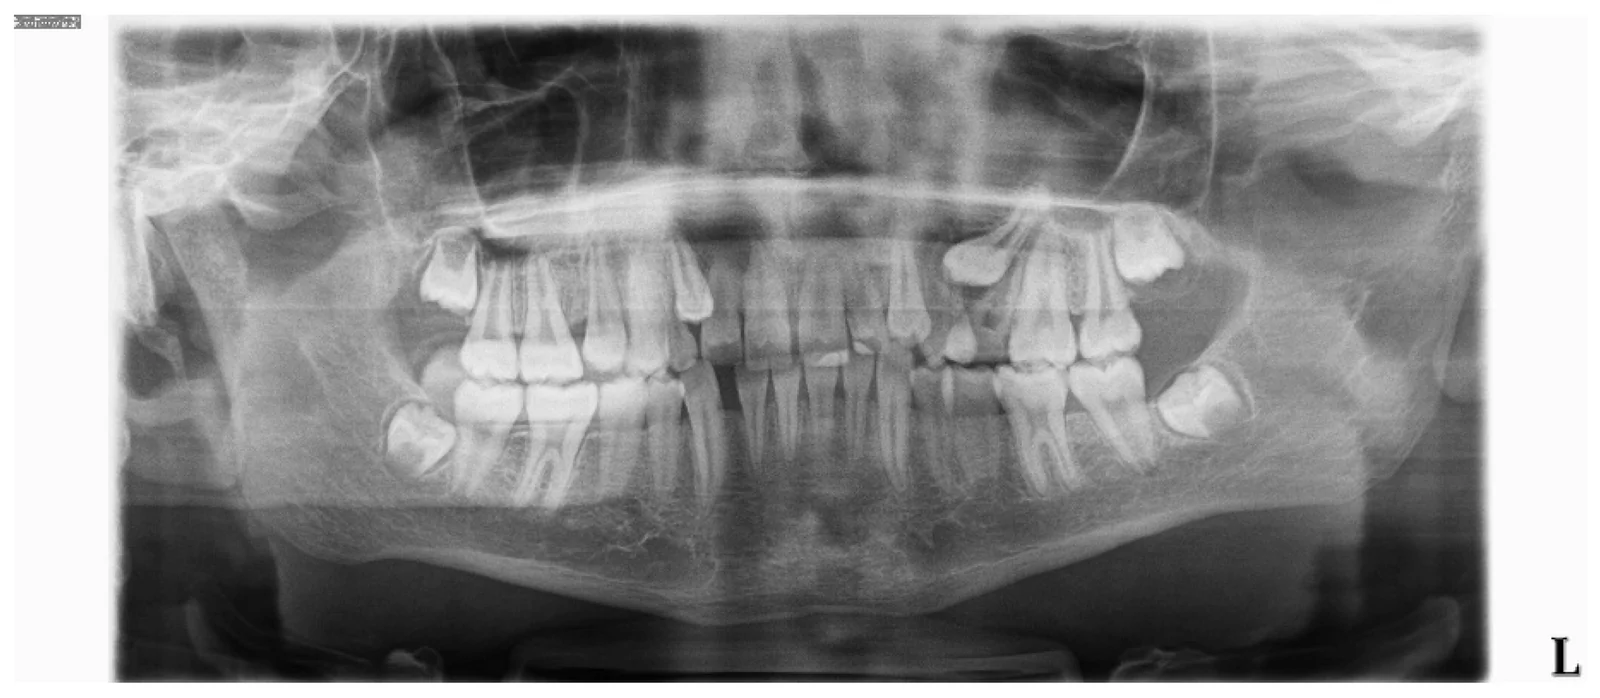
A panoramic radiograph from a 15-year-old girl demonstrating the coexistence of hypotaurodontism in the maxillary permanent first molars and pyramidal molar morphology in all permanent second molars.
